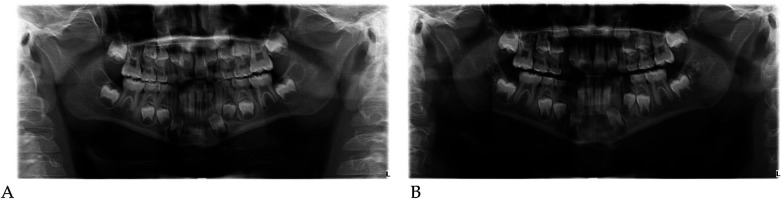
Pre- (A) and post-treatment (B) panoramic radiographs of case 2.
